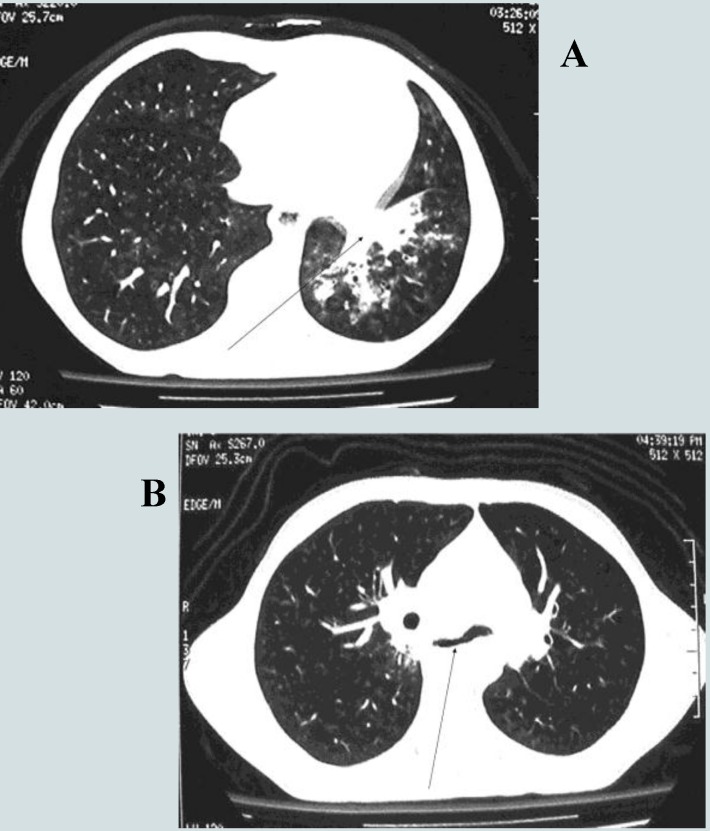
Thoracic CT scan: A - left lower lobe pneumonia; B – left eso-bronchial fistula
